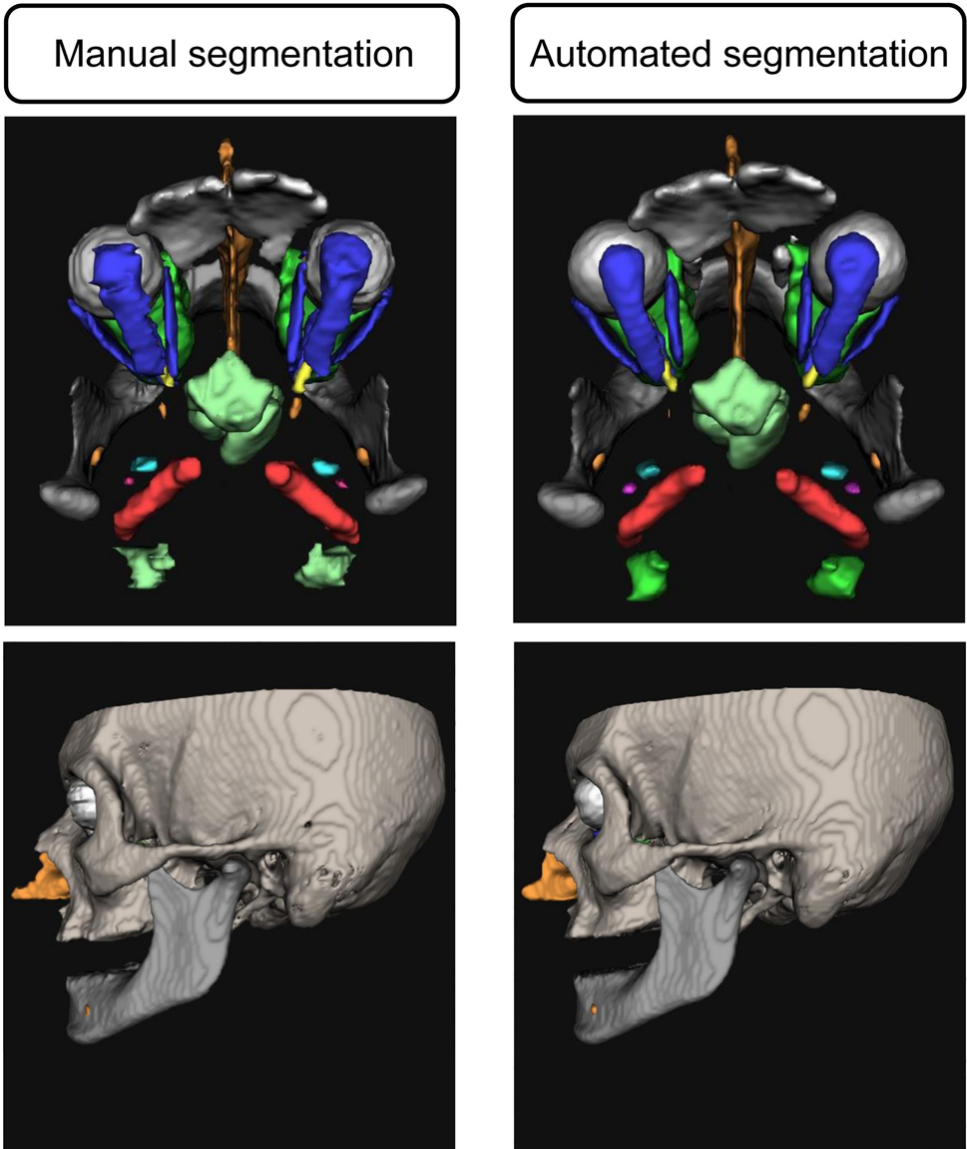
3D reconstructions of results of manual and automated segmentation from a CT scan of the validation dataset
